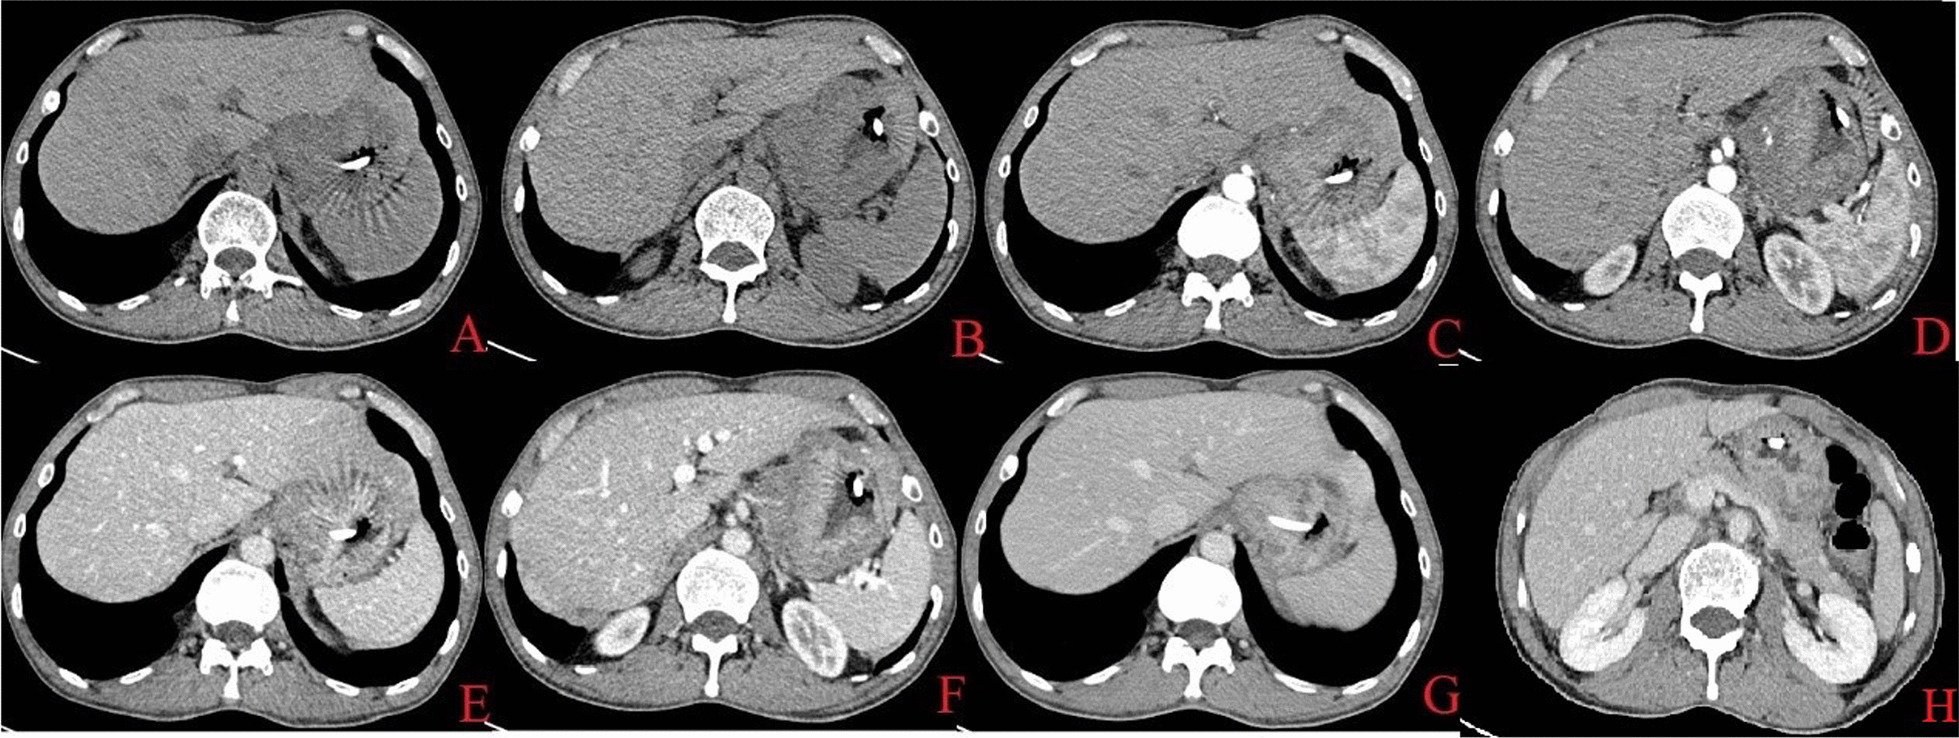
Enhanced CT images of the patient at the current admission (Siemens Force) CT plain and enhanced scan. Planes A, B plain scans, C, D arterial phase, E, F the portal vein phase, G the substantial phase, H image of extrahepatic bile duct. The indwelling nutritional tube shadow was seen in the stomach and esophagus. The thickening of the stomach wall was obvious, especially in the fundus and lesser curvature. On contrast-enhanced scan, the lesions showed heterogeneous enhancement. No obvious enlarged lymph nodes were found in the fat space around the lesion. There were no obvious dilatations of intra- and extrahepatic bile ducts. Imaging diagnosis showed that the gastric wall of the cardia, fundus, and stomach body were thickened, suggesting a tumor
